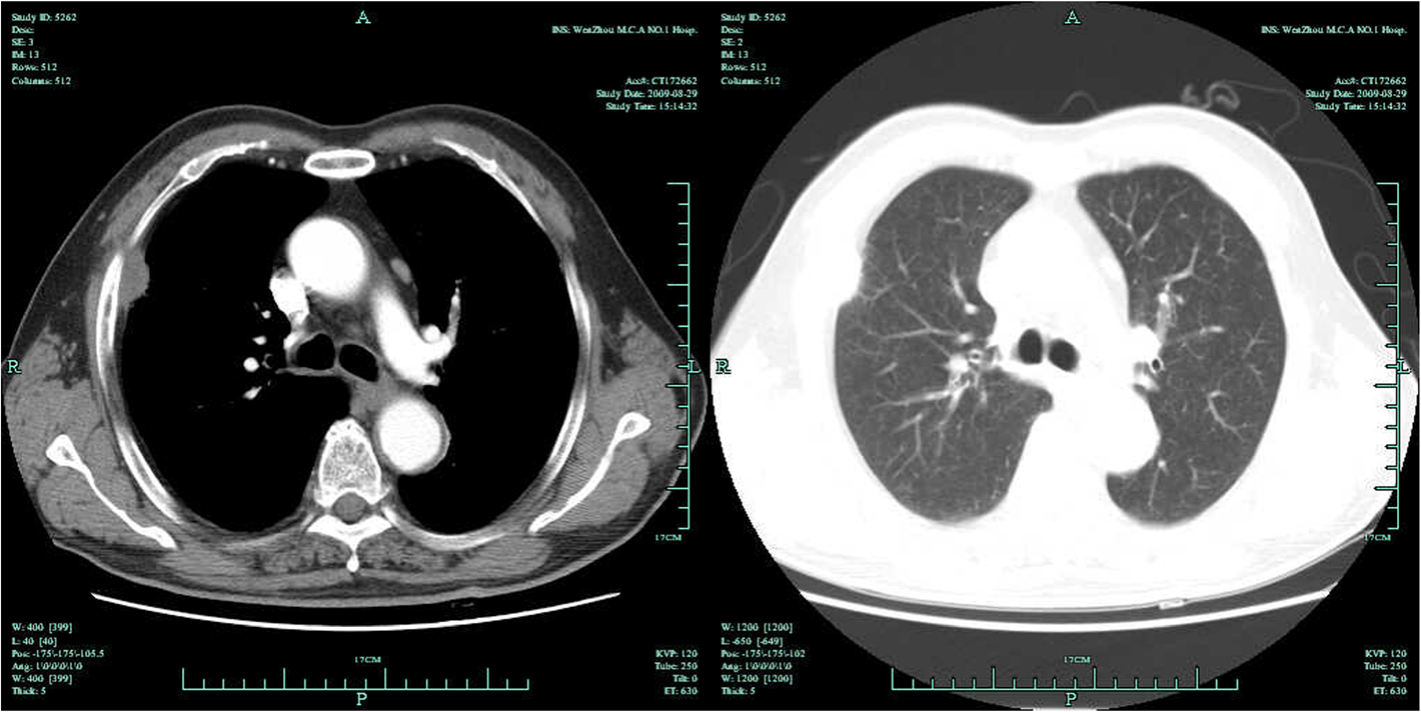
First chest CT scan on 29 August, 2009.
